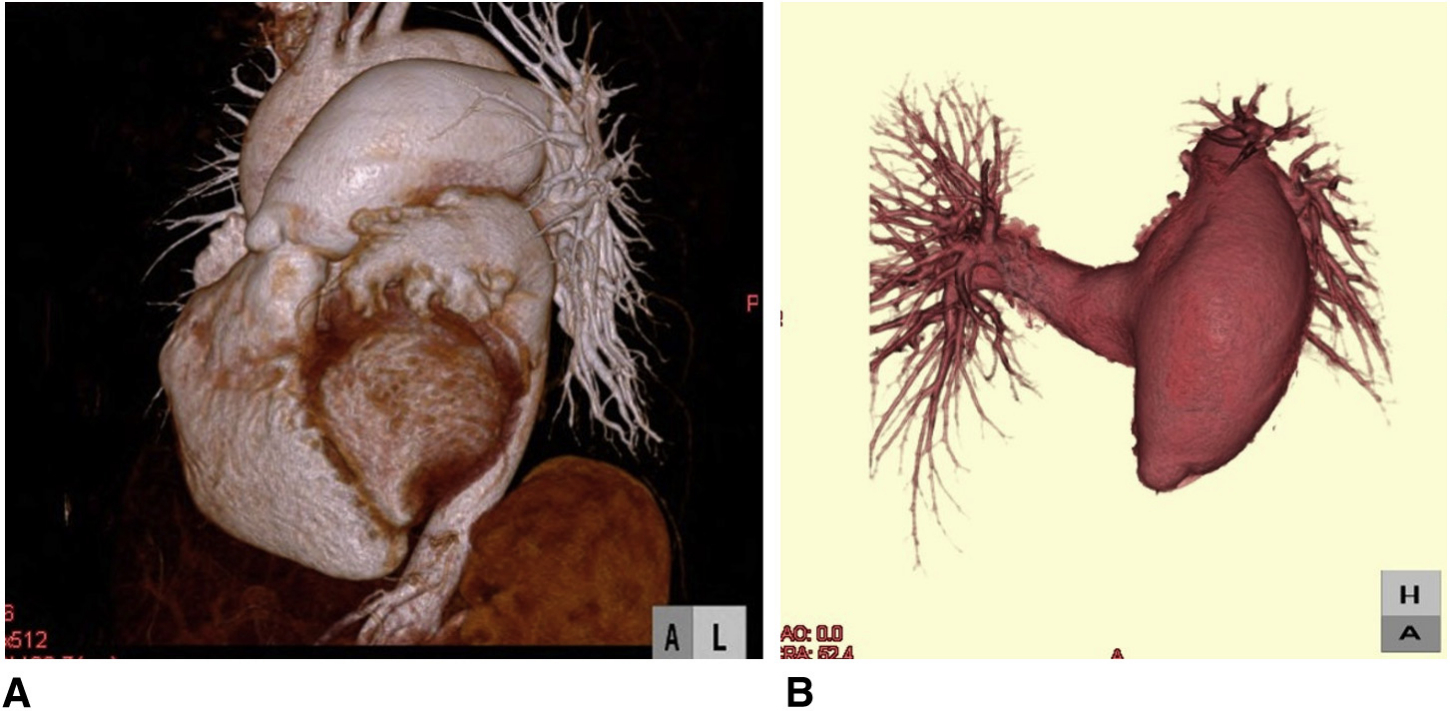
A and B, Preoperative computed tomography. The main pulmonary artery, right pulmonary artery, and left pulmonary artery are dilated.
